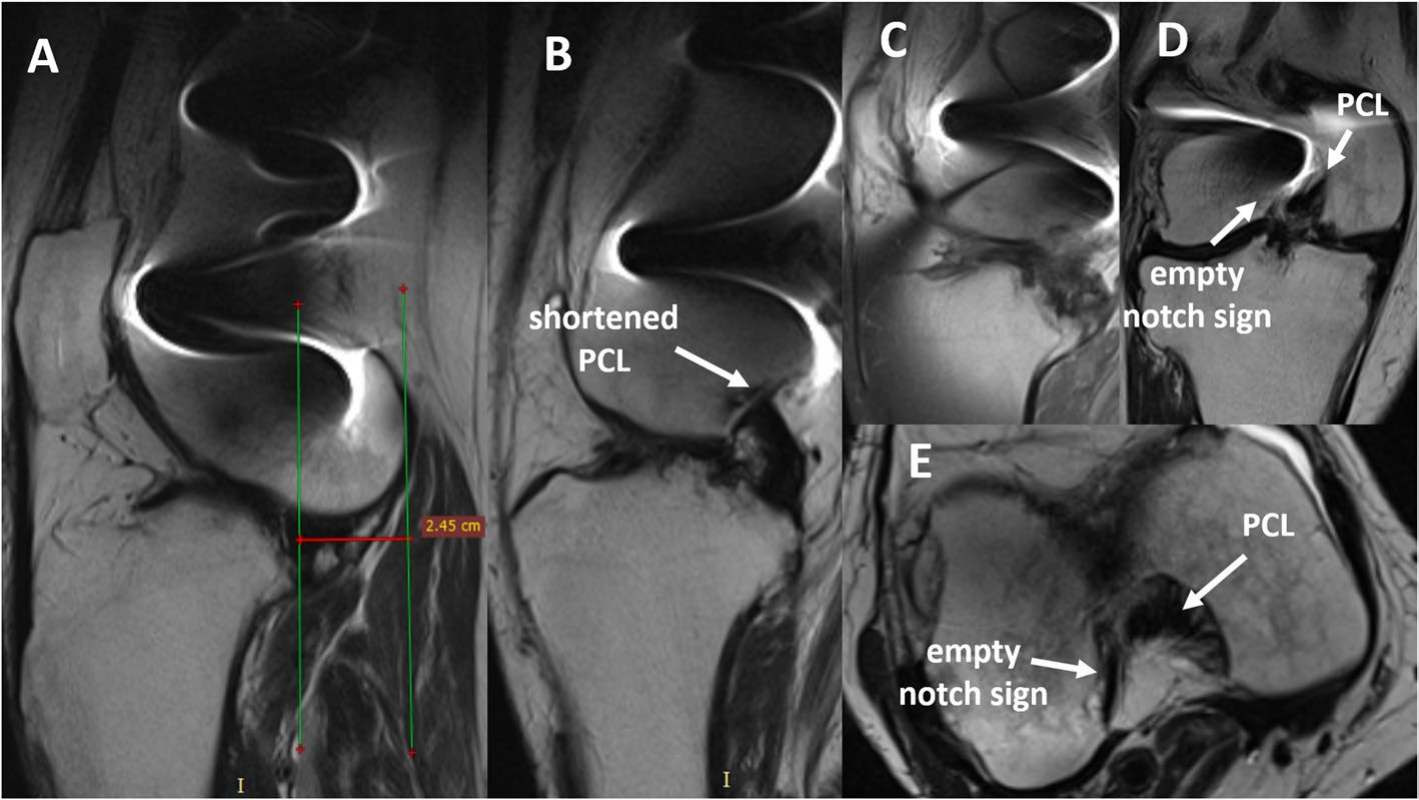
MRI at initial presentation to authors’ clinic. Anterior tibial subluxation (A) and PCL shortening (B) can be seen. No ACL fibers could be observed on sagittal (Fig. 2B, 2C), coronal (Fig. 2D) nor axial scans (Fig. 2E), in accordance with existing fixed anterior tibial knee subluxation. *ACL* Anterior cruciate ligament*, PCL* Posterior cruciate ligament
